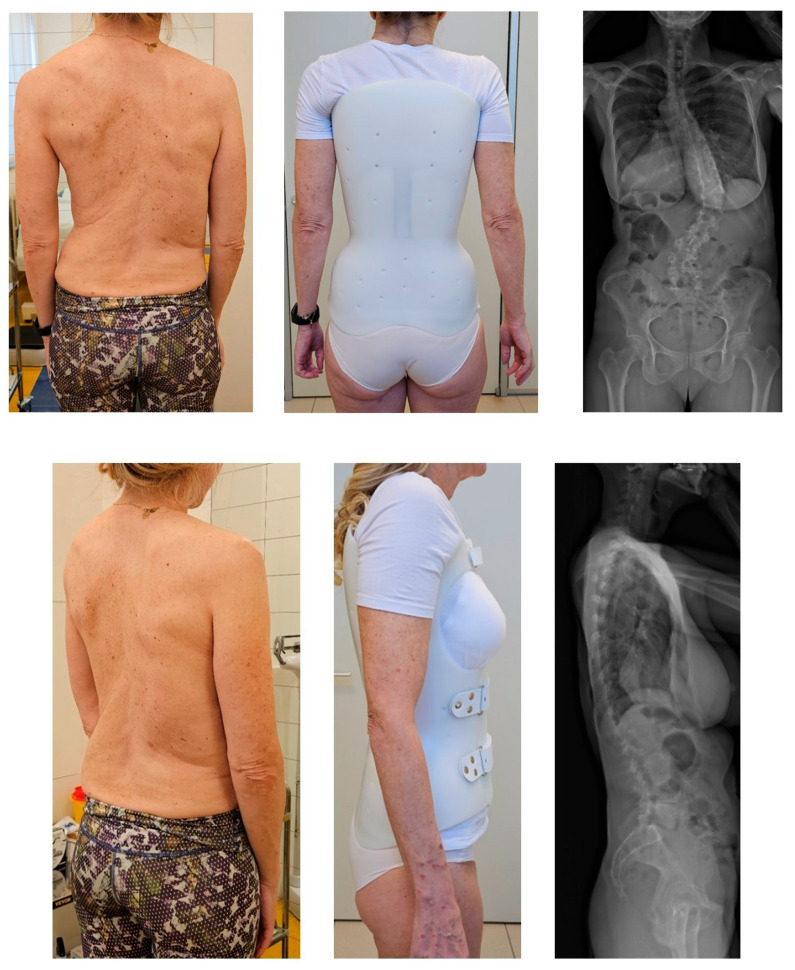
A patient at baseline, wearing a custom-made brace and her x-ray. Frontal (upper part) and lateral (bottom) view. On the left side, the trunk appearance at baseline. In the middle, the brace on the day it was delivered to the patient and checked by the treating physician and the orthotist. On the right, the most recent out-of-brace X-ray (EOS System) taken one month before the brace was built and delivered.
